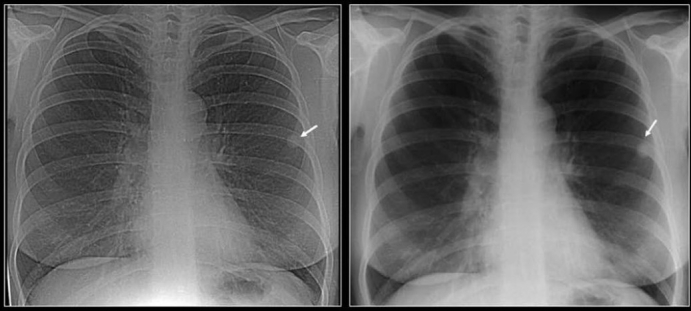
Two chest radiographs 5-years apart showing a high-density solitary pulmonary nodule remaining unchanged over a 5-year period. One of the most reliable imaging features of a benign lesion is as a benign pattern of calcification and periodic follow-up with CT showing no growth for 2 years. The high density of the well-defined nodule suggest that this is calcified granuloma and no further follow-up is indicated except in patients with calcium producing tumors such as a primary osteosarcoma
